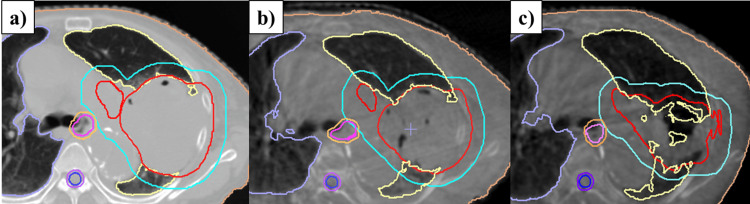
Adapt Anatomy on CBCT images. (a) Initial plan contouring. (b) Automatic contouring using Adapt Anatomy at the time of 20 Gy CBCT and (c) 40 Gy CBCT. The GTV is shown in red, the PTV in light blue, the esophagus in magenta, the spinal cord in blue, the left lung in light yellow, and the right lung in light purple. CBCT: cone-beam computed tomography; GTV: gross tumor volume; PTV: planning target volume
